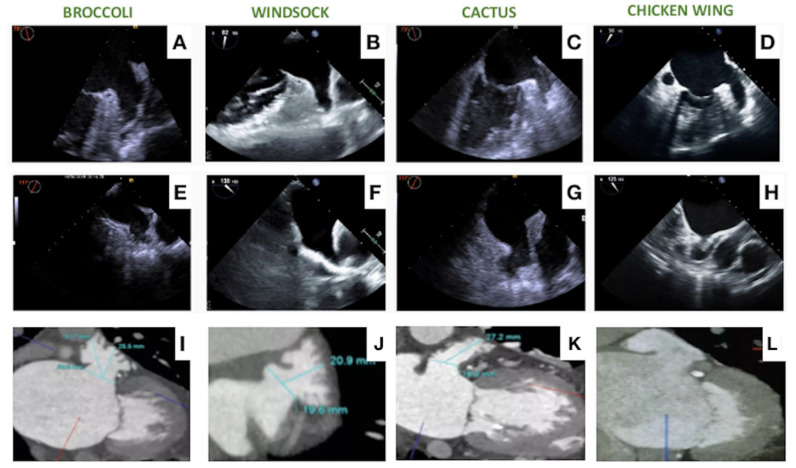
Most common LAA anatomies as visualized by TEE and CT, i.e., Broccoli (A,E,I), Windsock (B,F,J), Cactus (C,G,K), Chicken Wing (D,H,L).
